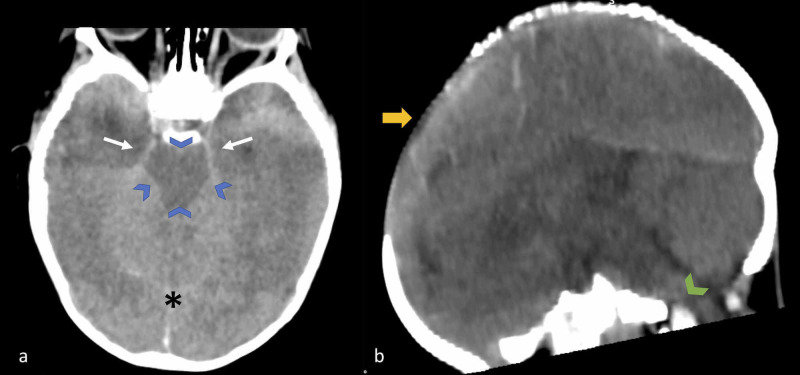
Brain CT scan of a nine-month-old child with fulminant brain edema. (a) Axial view: diffuse brain edema, obliteration of the peri-mesencephalic cisterns (blue arrowheads), temporal lobes edema without herniation (white arrows), and obliteration of superior cerebellum cistern (asterisk). (b) Coronal view: diffuse brain edema with protrusion of anterior fontanelle (yellow arrow) and collapse of pre-pontine cistern (green arrowhead). CT, computed tomography
